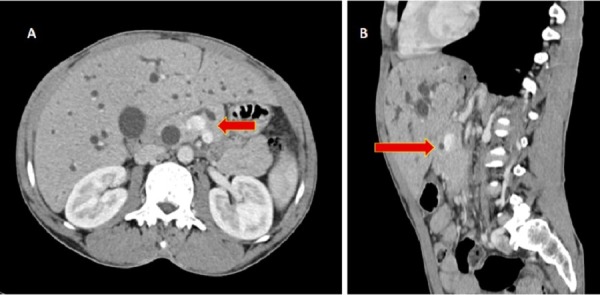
The coronal (A) and sagittal (B) views of the preoperative contrast enhanced computed tomography (CECT) scan demonstrating circumportal pancreas. The main pancreatic duct is anteportal.
